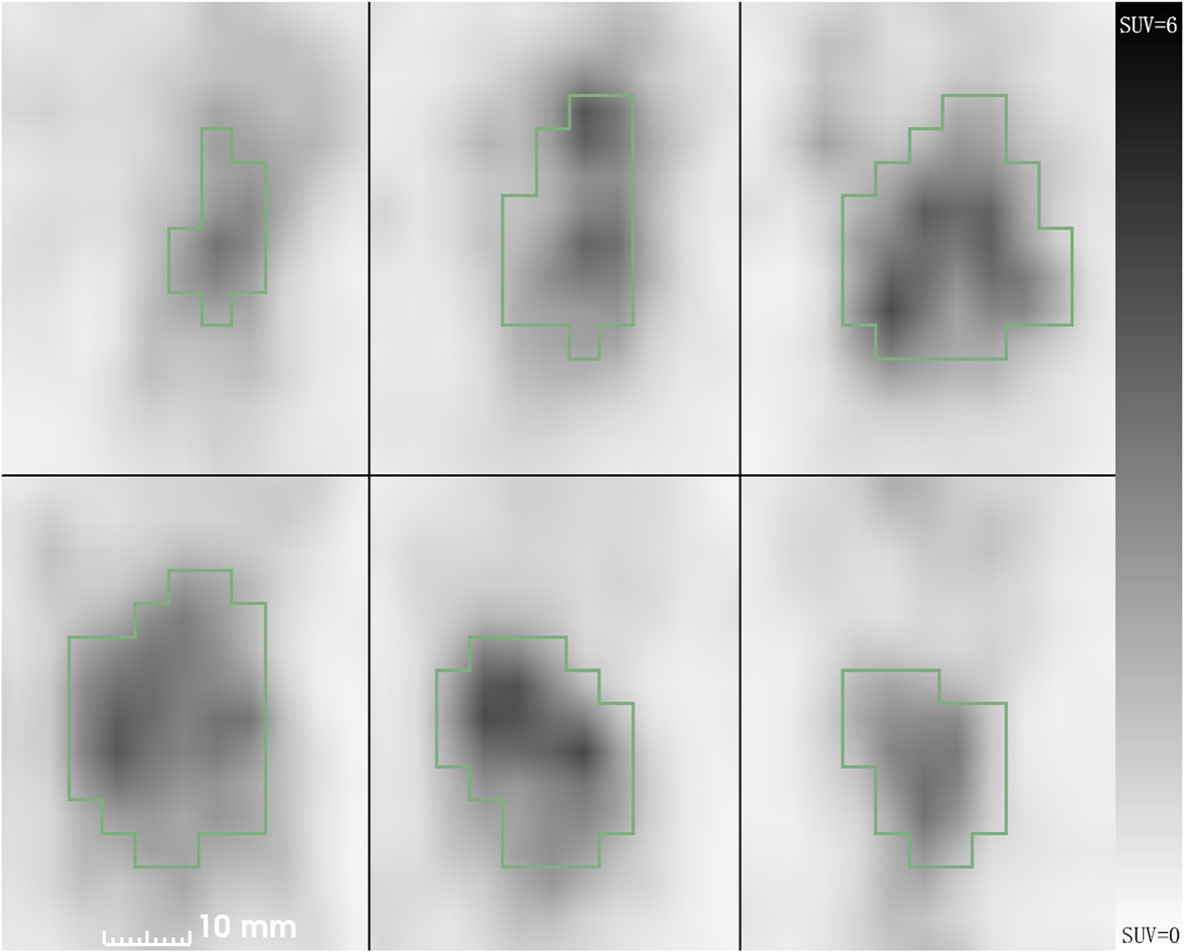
Delayed PET images of a reduced nodule are shown. The high uptake regions of the lesion are separated spatially, which leads to a higher busyness value and a lower coarseness value (delayed SUVmax = 5.80, delayed busyness = 5.98*10^−2^, delayed coarseness = 4.41*10^− 2^, delayed cluster prominence = 2.65*10^8^)
